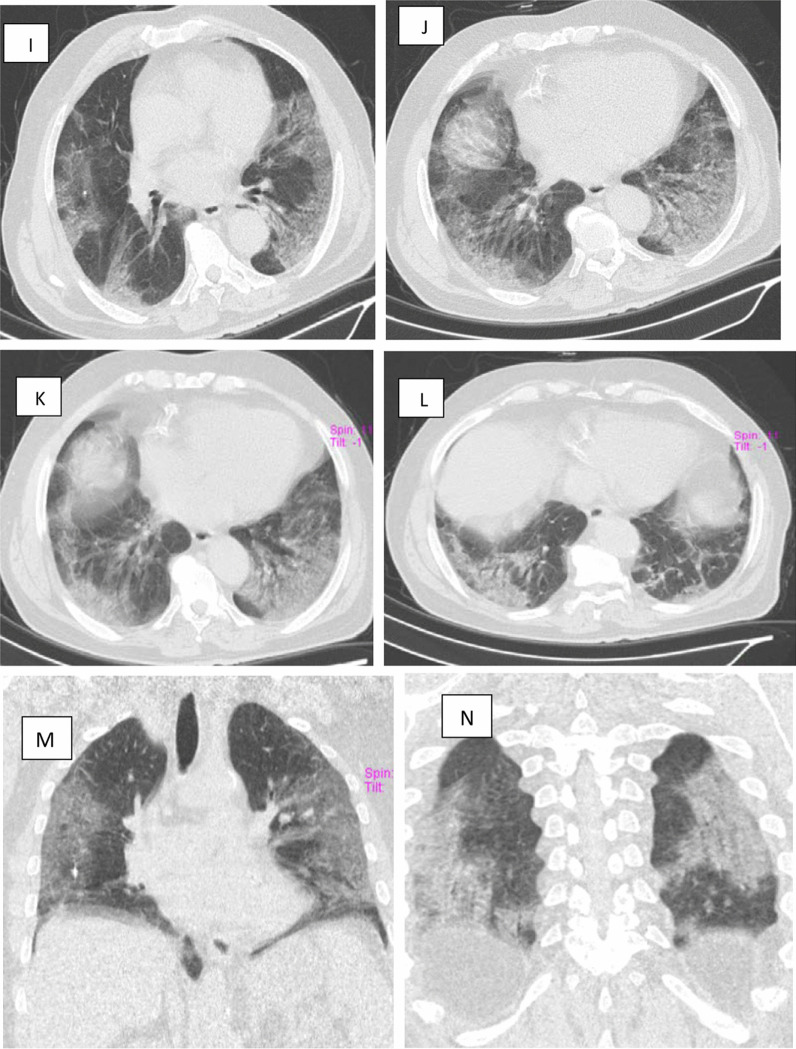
A 61-year-old infected nurse with COVID-19 presented with dyspnea, cough and decreased O2 saturation level to 83 percent. A–C Axial chest CT cuts (lung window) showing multiple bilateral sub-pleural patchy areas of ground glass attenuation and sub-segmental consolidations in both apical segments of right and left upper lobes (5% and 35%, respectively). C–H Axial chest CT cuts (lung window) showing involvement of anterior segments of both upper lobes and apical segments of both lower lobes (25–50%). I–L Axial chest CT cuts (lung window) showing involvement of lingula, middle lobe and both right and left lower lobes (10–25% 35 & 75%, respectively) by patchy sub-segmental consolidative areas, curvilinear band and ground glass pattern. M and N. coronal cuts showing subpleural ground glass areas with sub-segmental sub-pleural consolidative patches. CT severity score (CTSS) is 14/25
